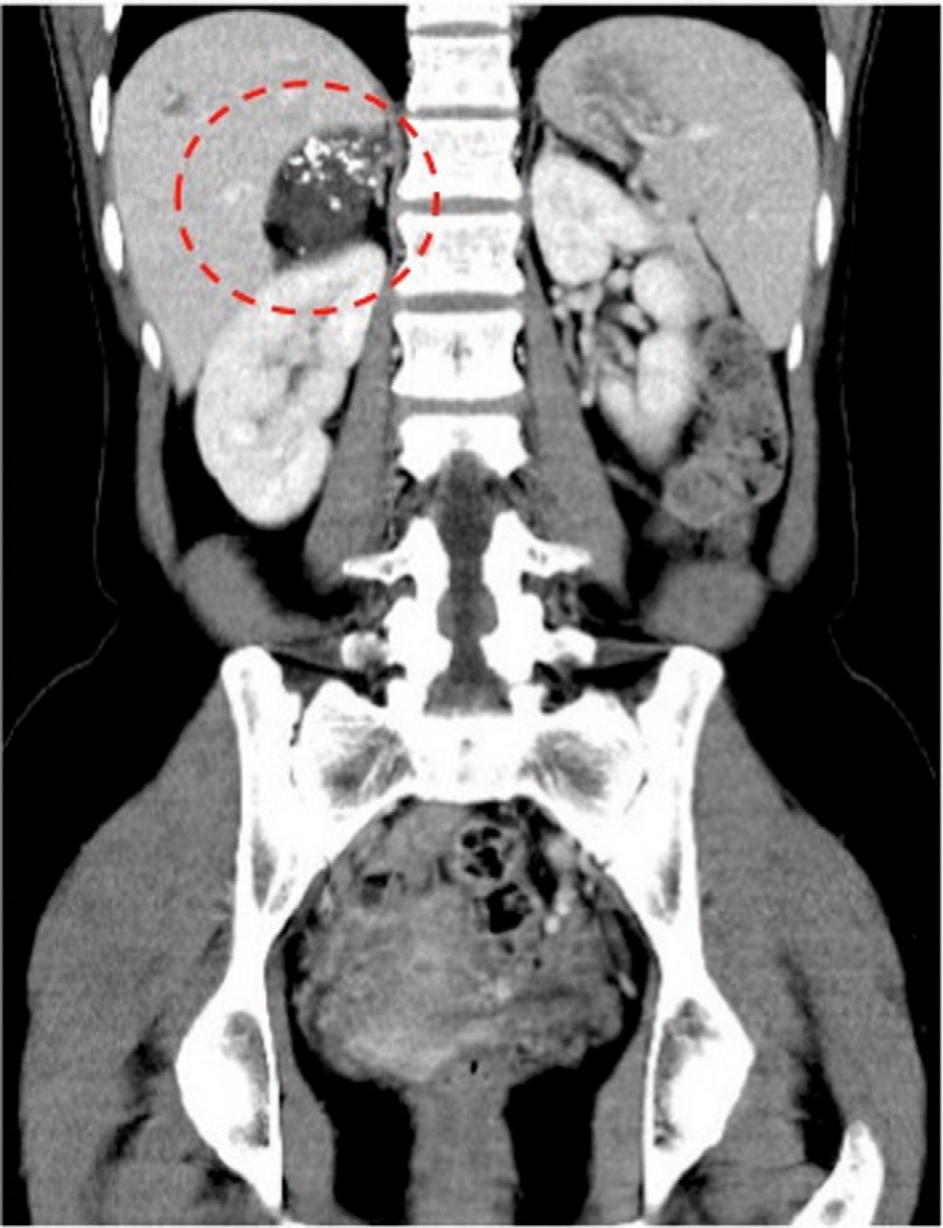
Contrast CT scan, coronal section. Right adrenal cystic tumor with calcifications shown in red dashed circle.
